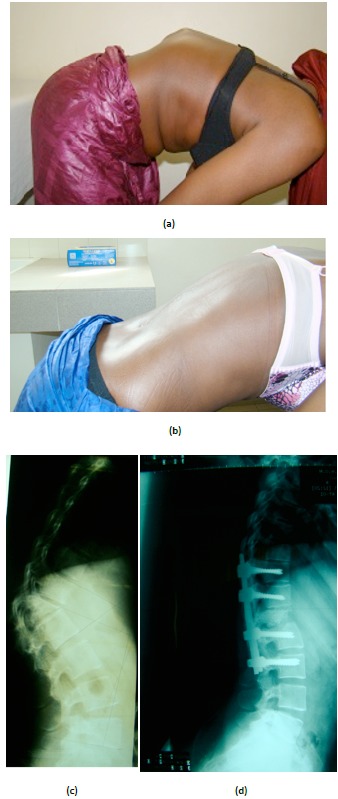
(a-b). Clinical pre et postopérative of patient N 4. (c-d). Xray pre et post operative of the same patient N 4.
